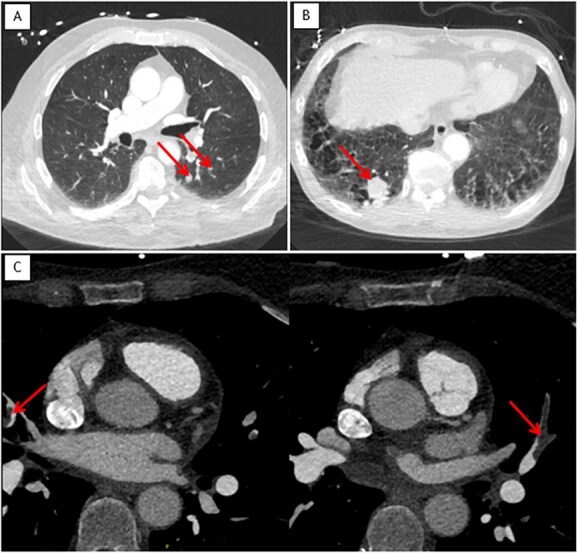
Example cases of non-cardiac incidental findings on cardiac-CT. Cardiac CT showing (arrows). (A) Two pulmonary nodules suspicious for malignancy of 11 and 7 mm in the left lower lobe. (B) A pulmonary mass of 25 mm suspicious for malignancy in the right lower lobe. (C) Bilateral segmental pulmonary embolism.
